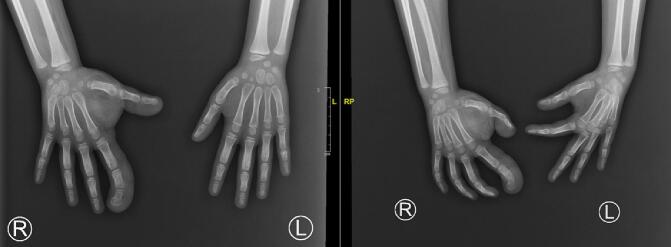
Plain radiographs of both hand before surgery.
